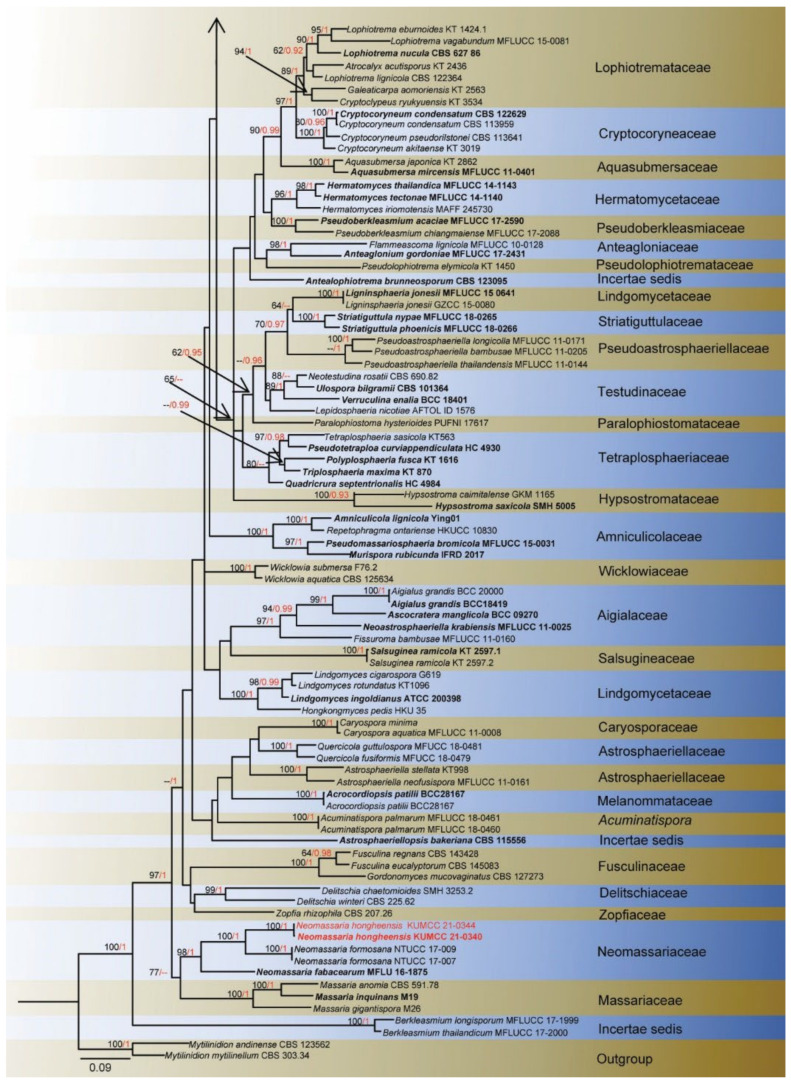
Phylogram generated from maximum likelihood analysis based on a combined LSU, SSU, *rpb2*, *tef1-α,* and ITS sequence datasets. Related sequences were taken from Zhang et al. [24], Ariyawansa et al. [30], and Hongsanan et al. [81]. The 180 strains are included in the combined gene analyses’ 4531 total characters, including gaps (LSU: 1–880 bp, SSU: 881–1913 bp, *tef1-α*: 1914–2840 bp, *rpb2*: 2841–3878 bp, ITS: 3879–4531), Tree topology of the ML analysis was similar to the BI. The matrix had distinct alignment patterns, with the final ML optimization likelihood value of −117,367.689956 (ln). All free model parameters were estimated by RAxML model, with 2953 distinct alignment patterns and 32.22% undetermined characters or gaps. Estimated base frequencies were as follows: A = 0.247310, C = 0.247387, G = 0.270304, T = 0.234998, with substitution rates AC = 1.384565, AG = 3.688216, AT = 1.401064, CG = 1.072637, CT = 7.214852, GT = 1.000000. The gamma distribution shape parameter alpha = 0.324973, and the Tree-Length = 19.839324. The final average standard deviation of split frequencies at the end of total MCMC generations was calculated as 0.009444 in BI analysis. The species determined in this study are indicated in red. Bootstrap values greater than 60% (ML, left) and Bayesian posterior probabilities (BI, right) greater than 0.90 are given at the nodes; hyphens (-) represent values less than 60% in ML/0.95 in BI.
